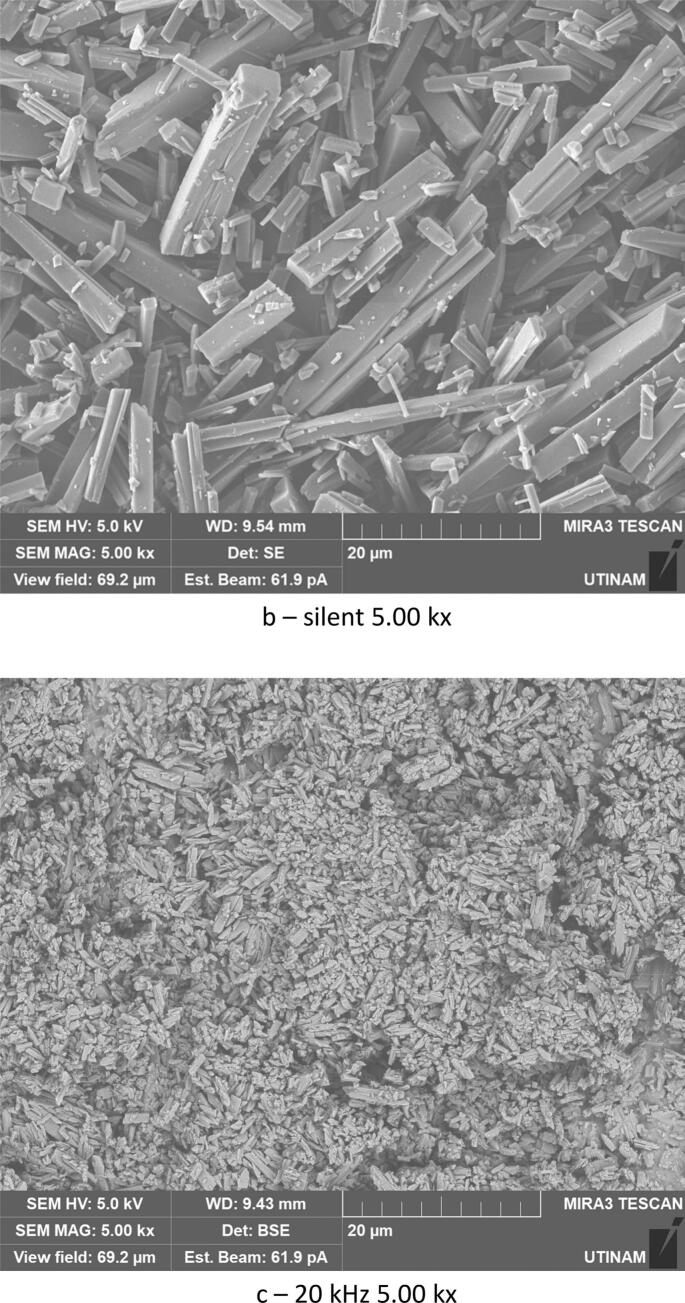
SEM analysis of crystals obtained under different treatment with the same magnification 5.00 kx after 10 min sonication/stirring (tests were carried out using the same concentration of 0.040 g/mL in sodium amoxicillin at pH 4.5 and using a power of around 7.25 W for the ultrasound treatment and a stirring speed of 100 rpm for silent conditions).
